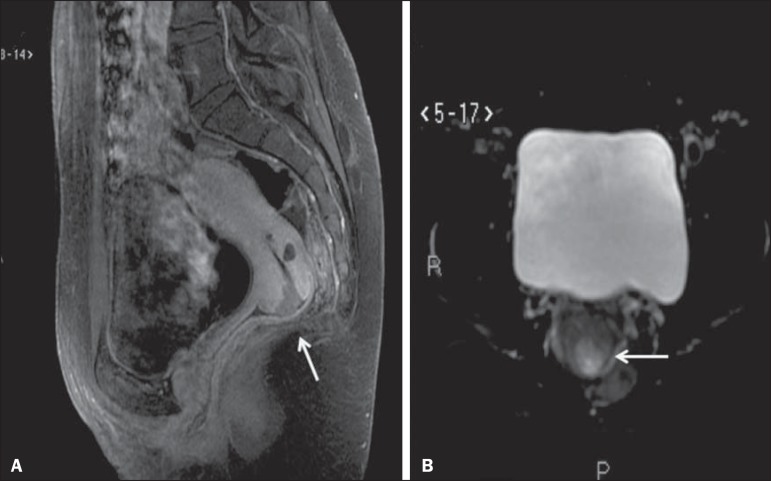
MRI scan requested for the detection/staging of a gynecologic neoplasm
